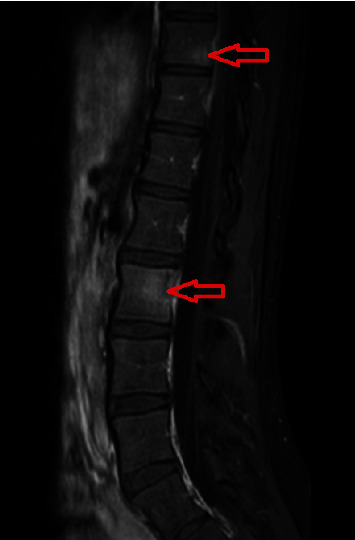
Increased signal intensity at the inferior endplate of the T10 vertebral body and at the posterior region of the L2 vertebral body (red arrows) in the contrast-enhanced T1-weighted sagittal lumbar spinal magnetic resonance imaging scan.
